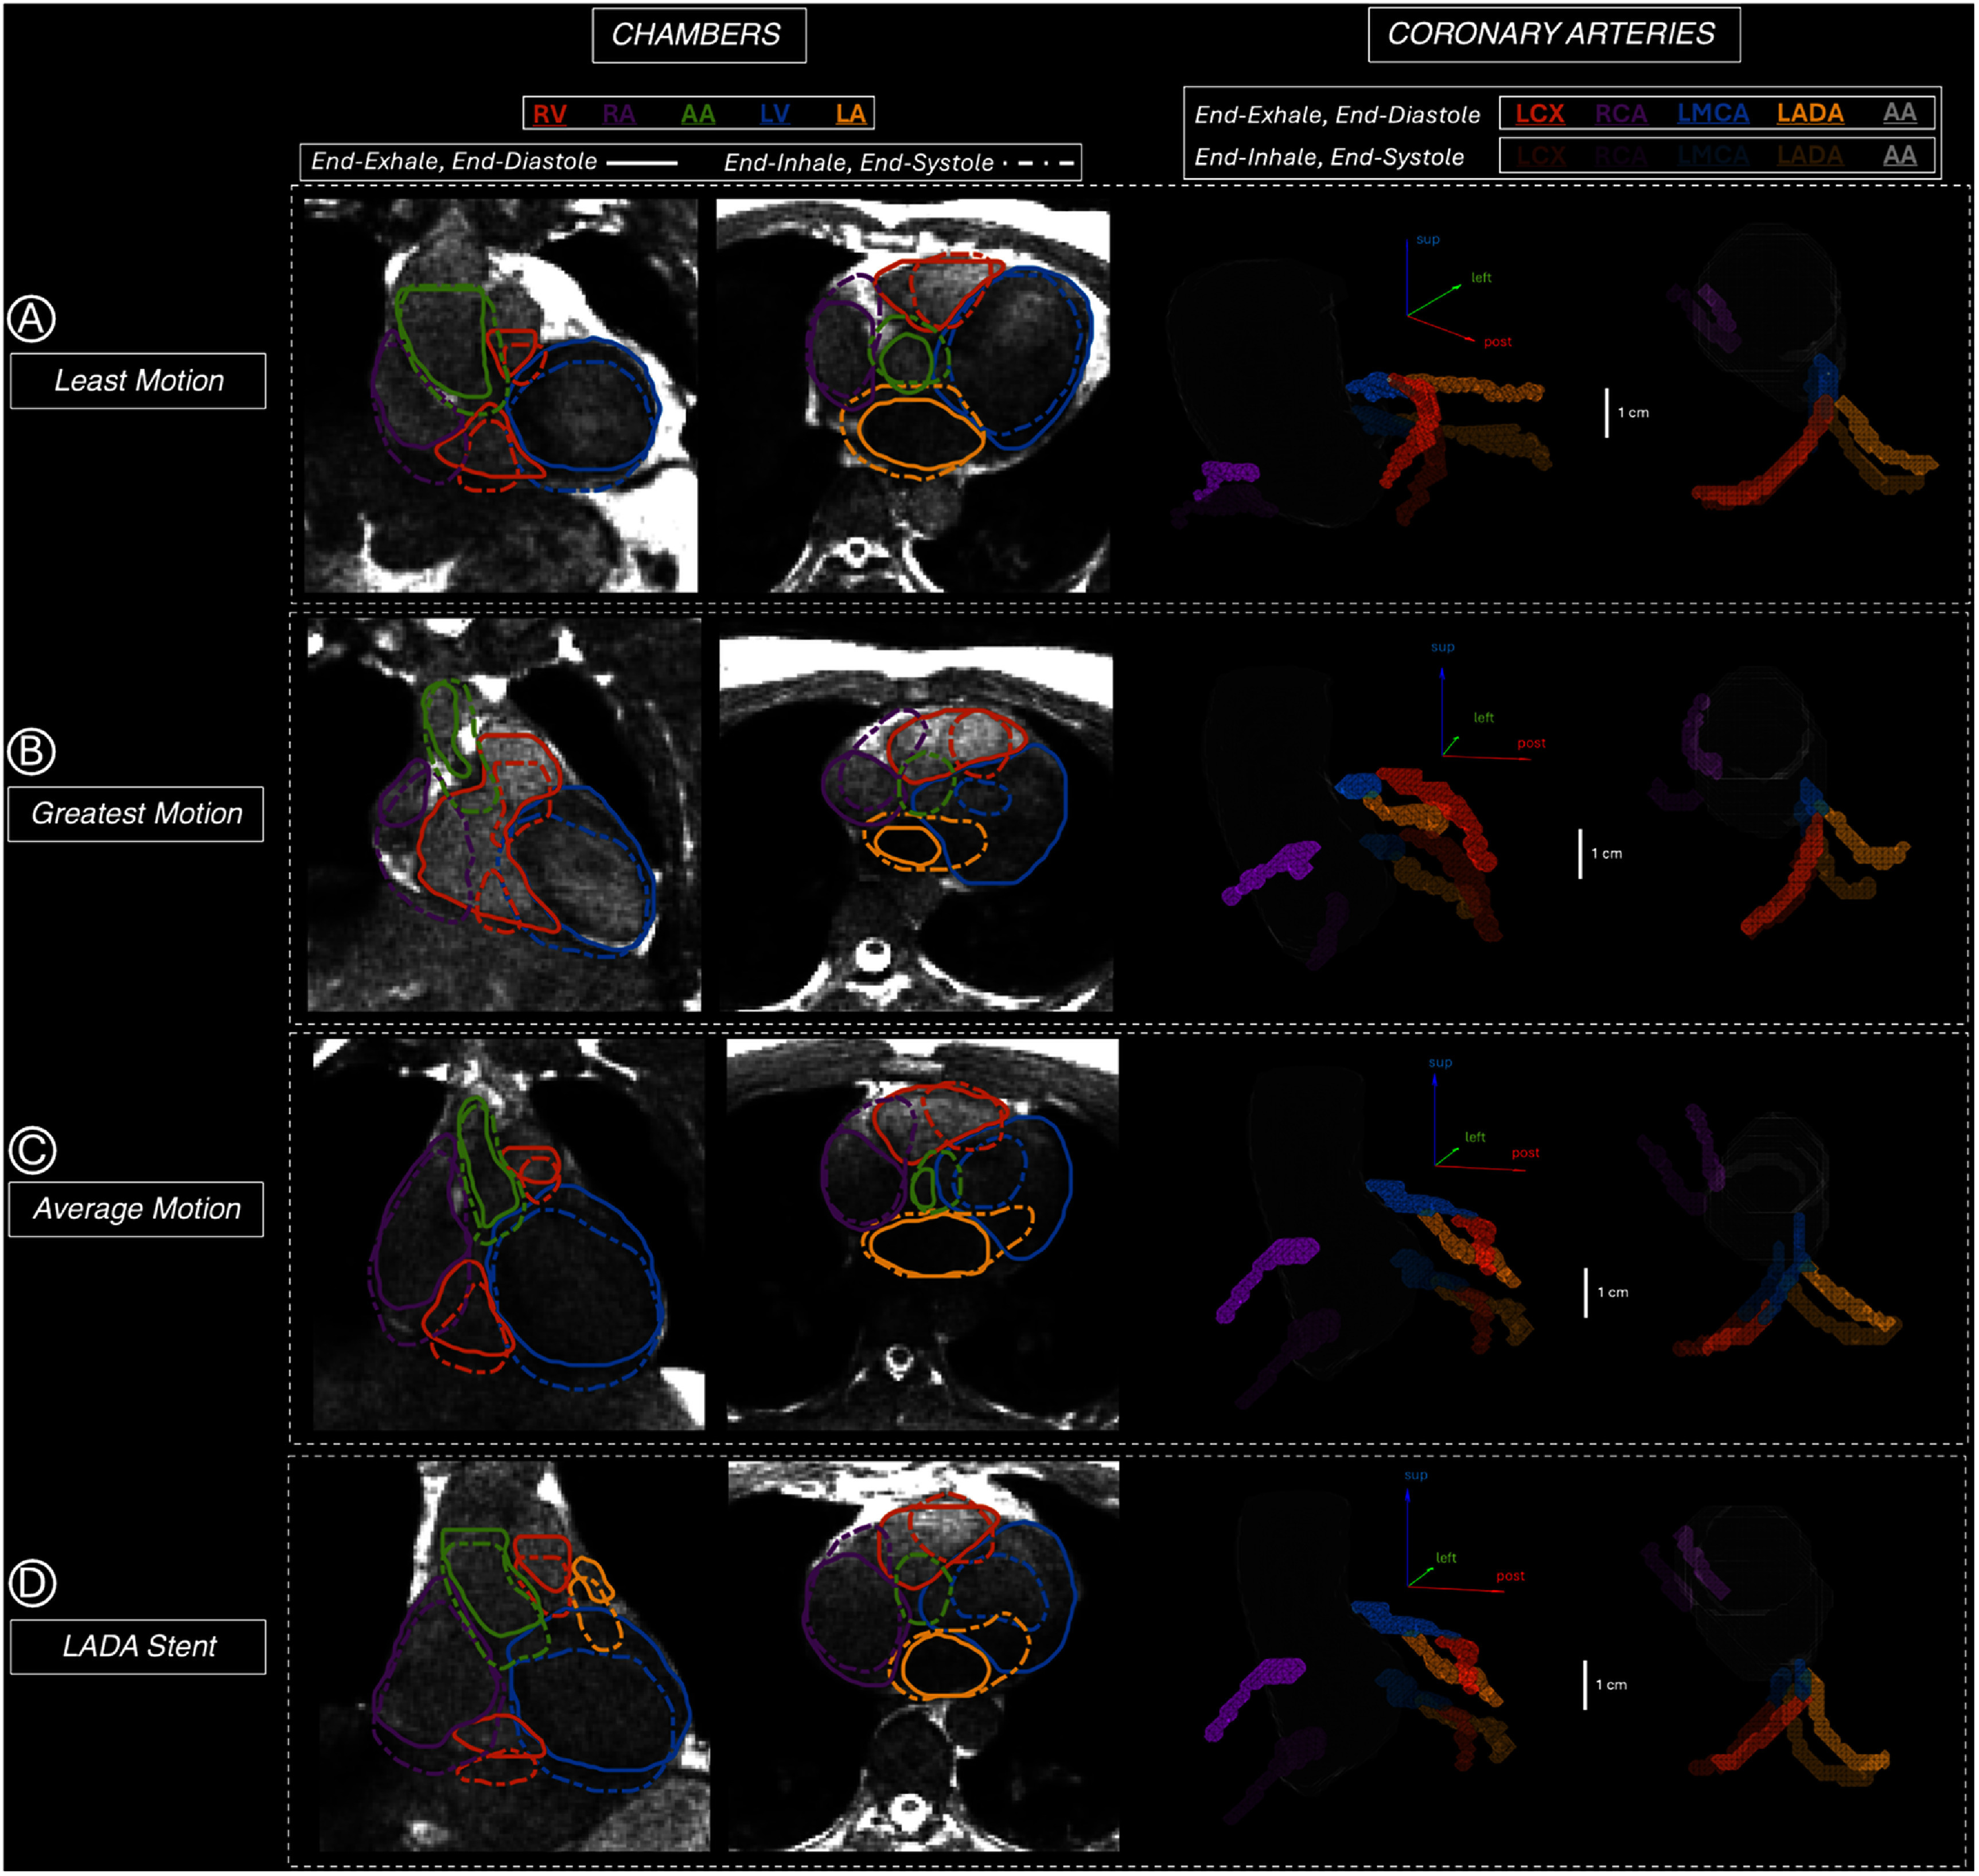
Contours for end-exhale, end-diastole (solid/bright) and end-inhale, end-systole (dashed/dark) on 5D-MRI are shown. Volunteers with the smallest (A), greatest (B), and average (C) magnitudes of cardiac motion, and a volunteer with an LADA coronary stent (D), are highlighted. Substructures generally move inferiorly, anteriorly, and left at end-inhale, end-systole compared to end-exhale, end-diastole. Locally, substructure displacements exceed 1 cm.
